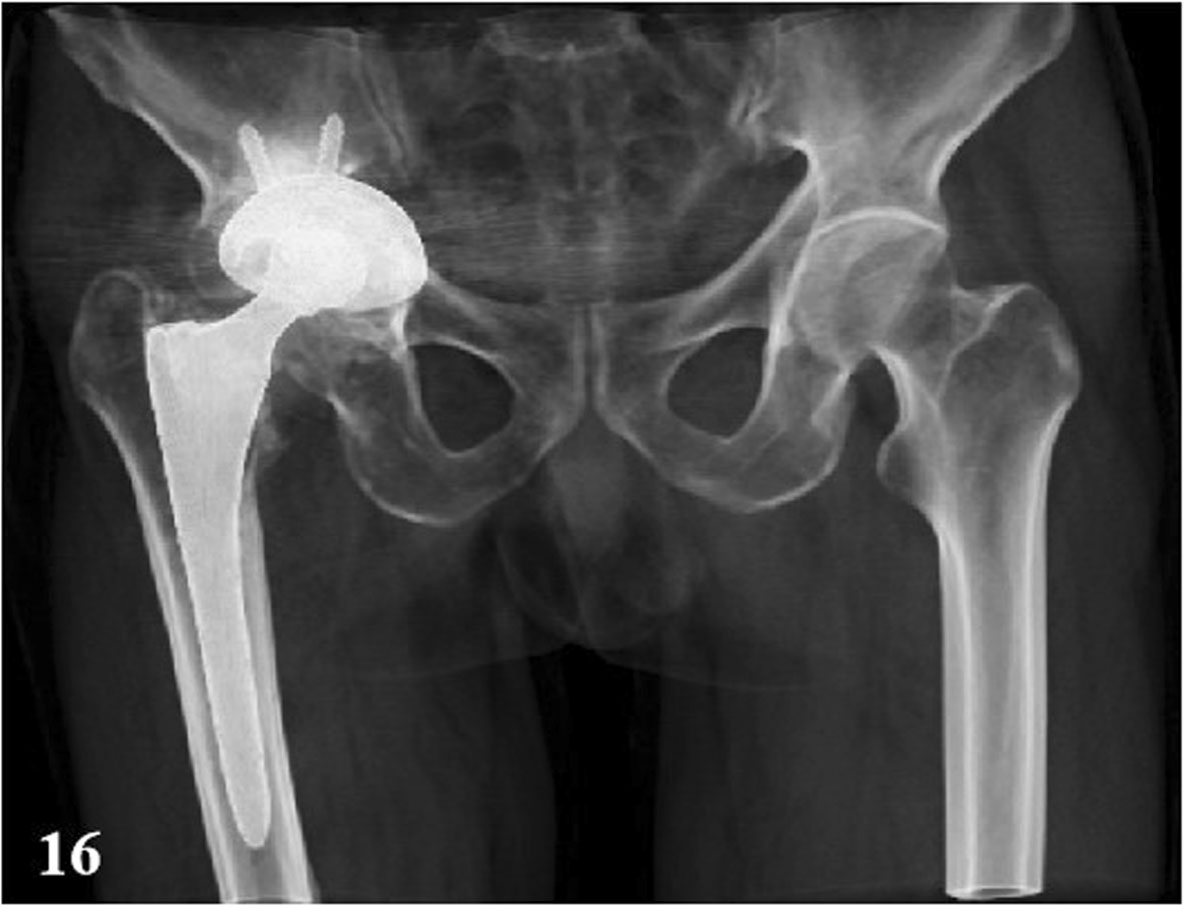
A AL patient’s volume-rendered 3D CT imaging
